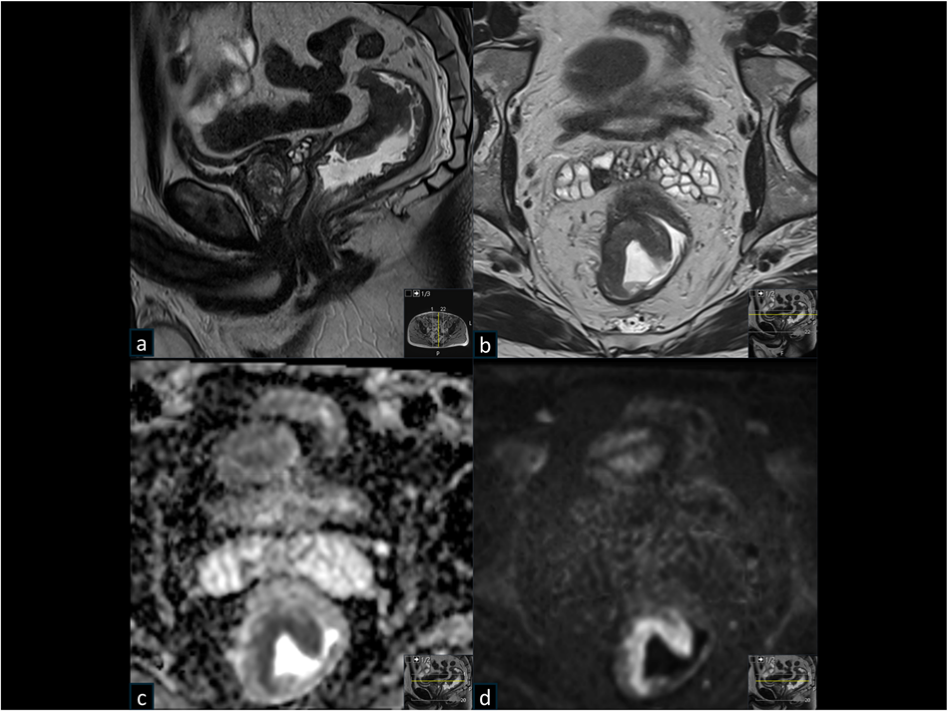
A 50-year-old man from OPT with PSA 4.00 µg/L. Incidental finding of semi-circumferential rectal tumour, which was operated (pT3bN0). T2W sag (a), T2W trans axial (b), ADC (c) and high b value DWI b1400 s/mm² (d)
